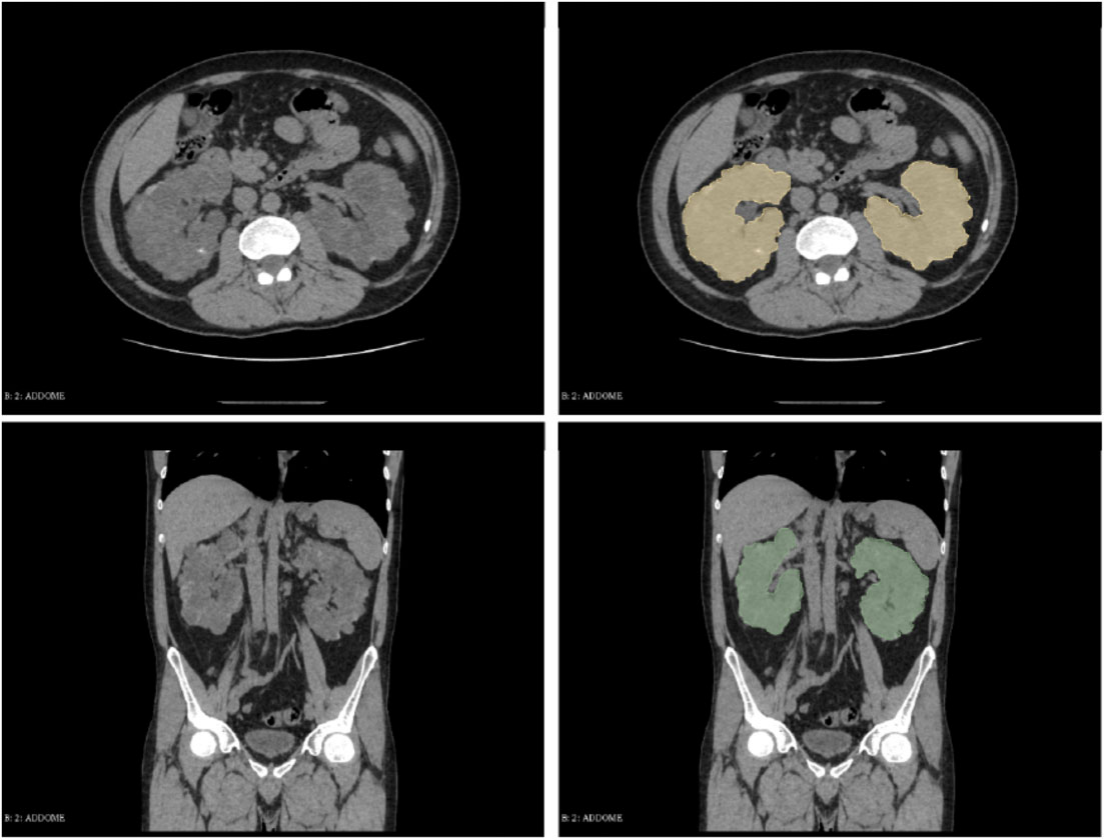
Axial and coronal CT images without intravenous contrast injection, before and after kidney segmentation. Note that the hilum of both kidneys was not included in the segmentation.
